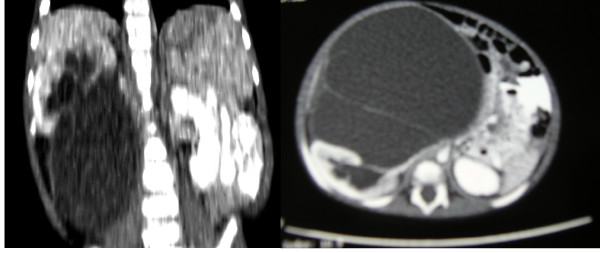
Axial/coronal computed tomographic scan of abdomen showing cystic, loculated mass inferior to right kidney.
